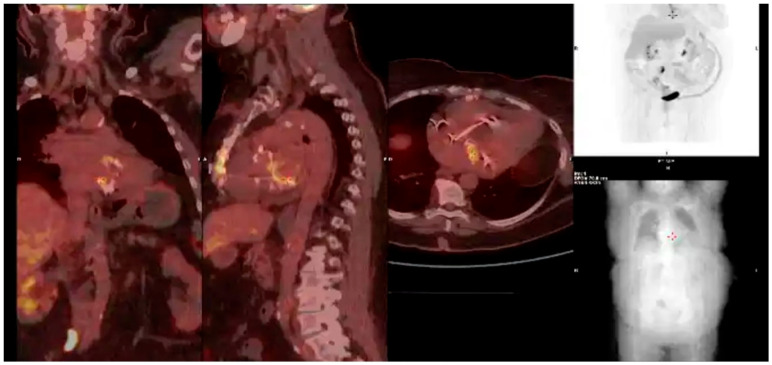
PET/CT findings in a patient with infective endocarditis involving a mechanical aortic prosthesis. This image shows tracer uptake at the level of the aortic prosthesis. This is an original, de-identified clinical image from our institution. Patient consent for publication was obtained.
